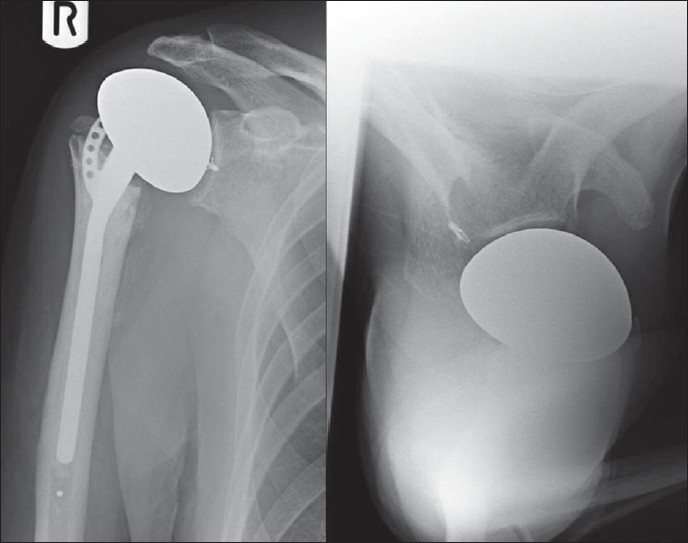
Postoperative radiographs of the right shoulder showing the prosthesis and the DePuy Mitek™ suture anchors *in situ*
